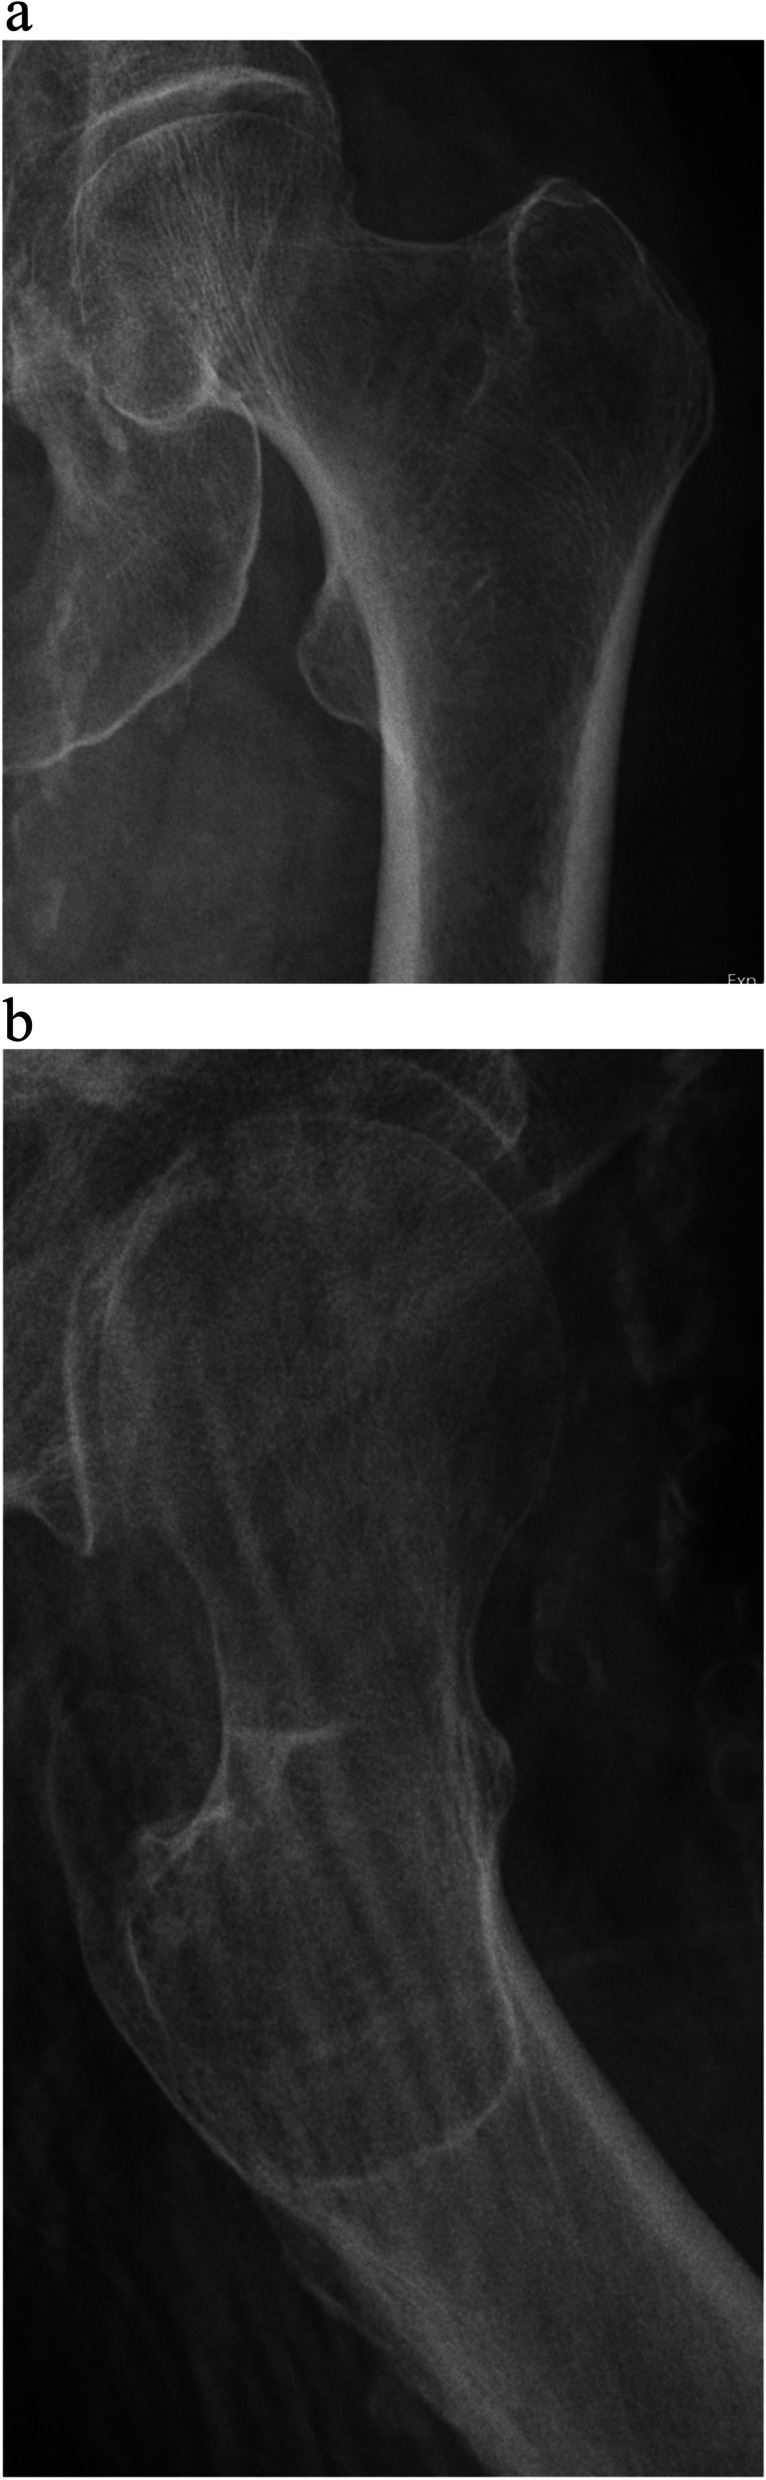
Plain radiographs of an occult femoral neck fracture. a) AP projection of the hip. b) lateral projection of the hip
